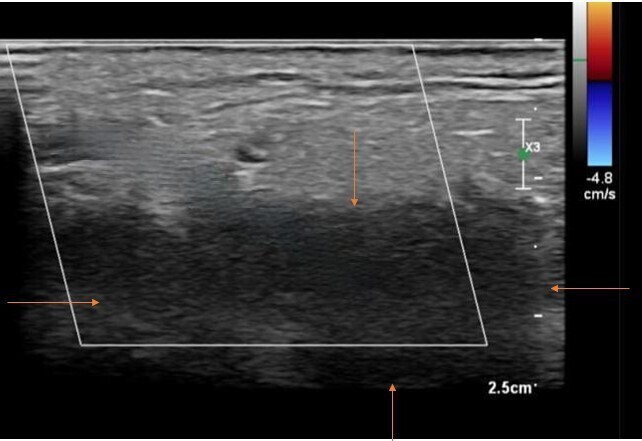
USG of right parotid gland showed a well defined irregular hypoechoic lesion in superficial and deep lobes (indicated by orange arrows) No significant vascularity is noted within the lesion in doppler study
